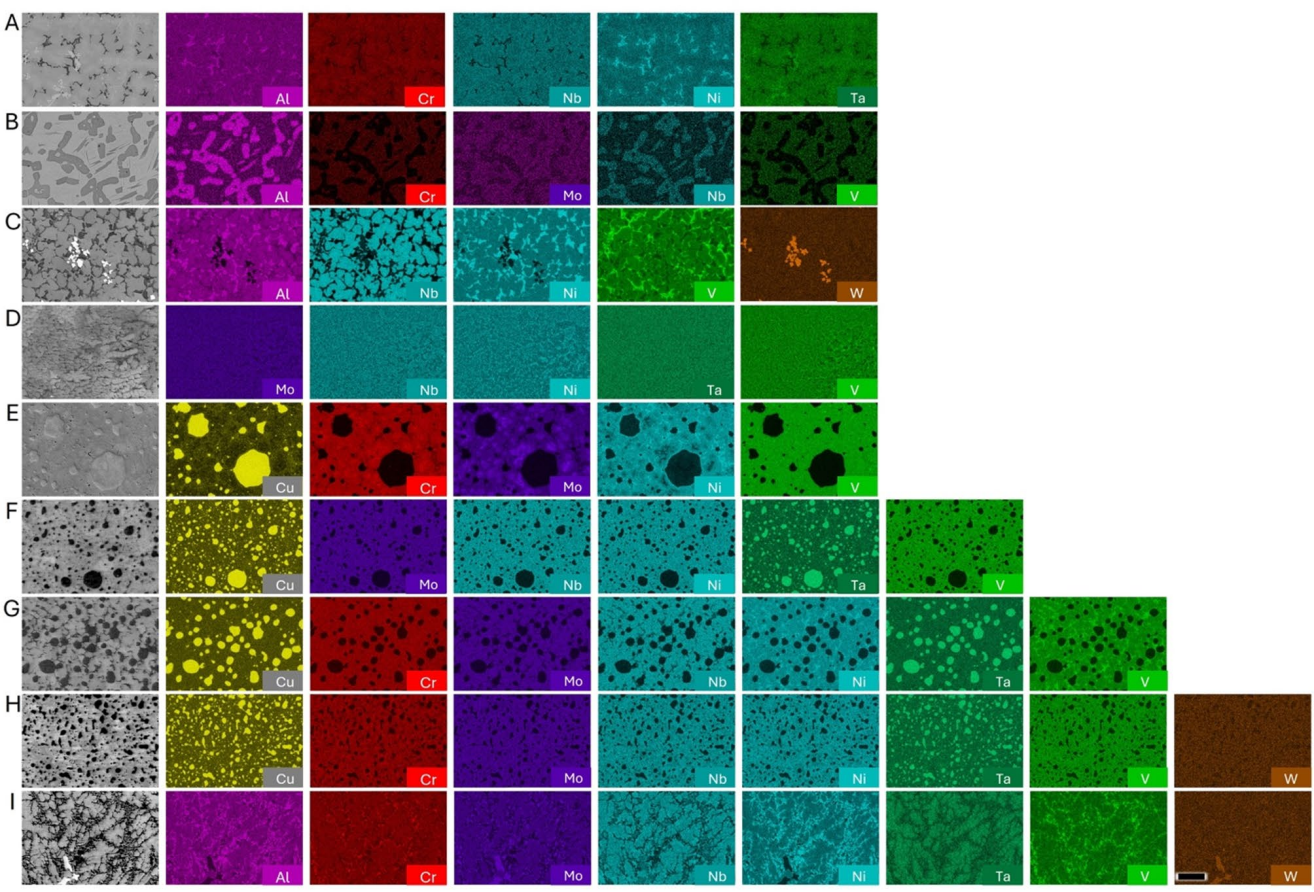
Characterization of fabricated compositions. An electron image is included with the EDS maps for (A) Al_8.90_Cr_11.45_Nb_20.45_Ni_19.37_Ta_39.83_ (B) Al_27.00_Cr_13.01_Mo_24.00_Nb_23.24_V_12.74_ (C) Al_8.87_Nb_20.37_Ni_19.30_V_11.17_W_40.30_ (D) Mo_14.64_Nb_14.17_Ni_35.81_Ta_27.60_V_7.77_ (E) Cr_13.61_Cu_24.93_Mo_25.10_Ni_23.03_V_13.33_ (F) Cu_19.11_Mo_14.42_Nb_13.97_Ni_17.65_Ta_27.20_V_7.66_ (G) Cr_6.68_Cu_20.41_Mo_12.33_Nb_11.94_Ni_18.85_Ta_23.25_V_6.54_ (H) Cr_5.08_Cu_18.65_Mo_9.37_Nb_9.07_Ni_17.21_Ta_17.68_V_4.98_W_17.96_ and (I) Al_8.87_Cr_5.69_Mo_10.50_Nb_10.17_Ni_19.28_Ta_19.80_V_5.57_W_20.12_. Each sample is observed to be multi-phase as arc melted. All compositions are provided in weight percent. Note that Ta and Cu have overlapping characteristic X-ray peaks. The scale bar in the lower right tungsten EDS map is 25 μm.
